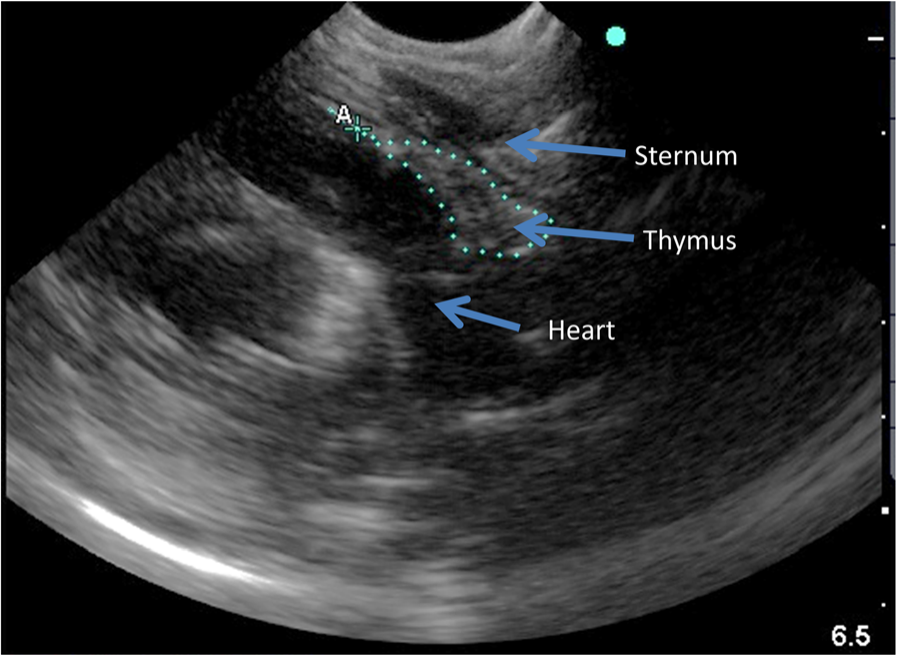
Ultrasound image of thymus in a malnourished child in the sagittal view. The line on the image traces the outline of the thymus, to measure the area
